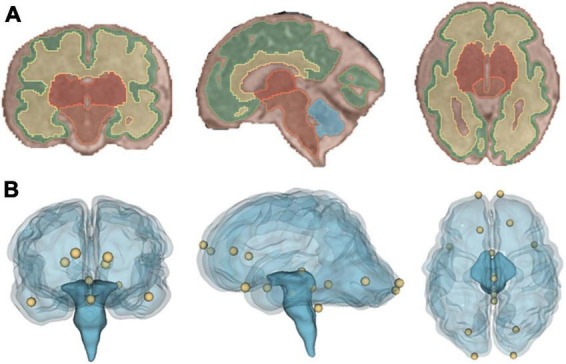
Visualization of the registration evaluation metrics used in our study. (A) Coronal, sagittal and axial view of segmentation overlaid on a T2-weighted SR image of a fetus. (B) Coronal, sagittal and axial view of a glass brain rendering showing all sixteen, manually placed landmarks that were used during the registration evaluation.
